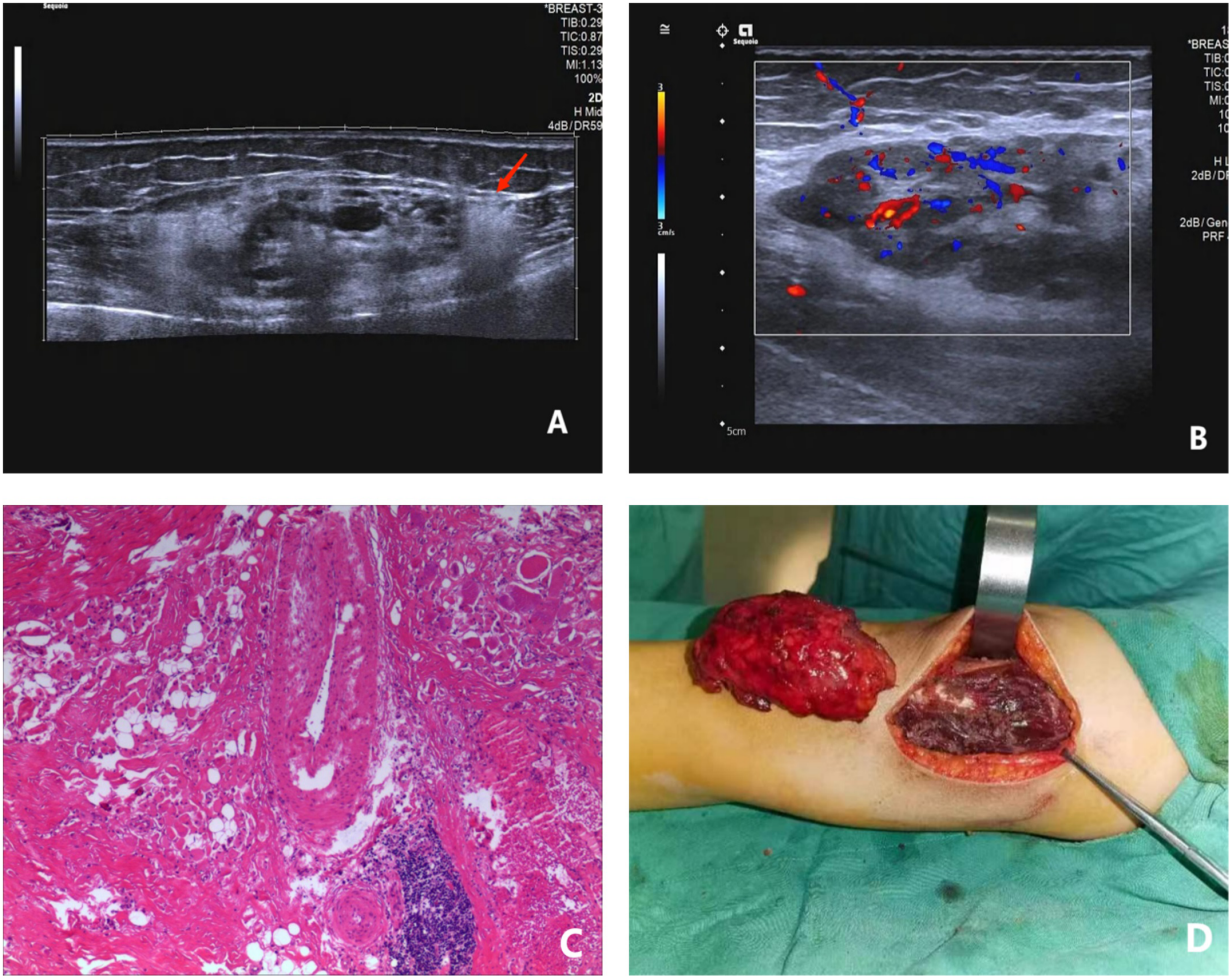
A female patient, 12 years old and suffering from intermuscular FAVA in the right thigh; (A) longitudinal 2D ultrasound image showed a mixed intermuscular solid mass with triangular hyperechoic “fascialtail sign” (red arrow) at the lower edge of the lesion; (B) through enlargement of local view of the lesion, CDFI suggested a small amount of blood flow signal within the lesion; (C) pathological findings showed existence of abnormally dilated vessels, fatty tissues and fibrous tissues in muscle cells. Fiber content could be found around the lesion, HE×100; (D) a bright red tumor with a tough texture was found during the operation in the deep layer of the right thigh muscle. FAVA, fibro adipose vascular anomaly.
